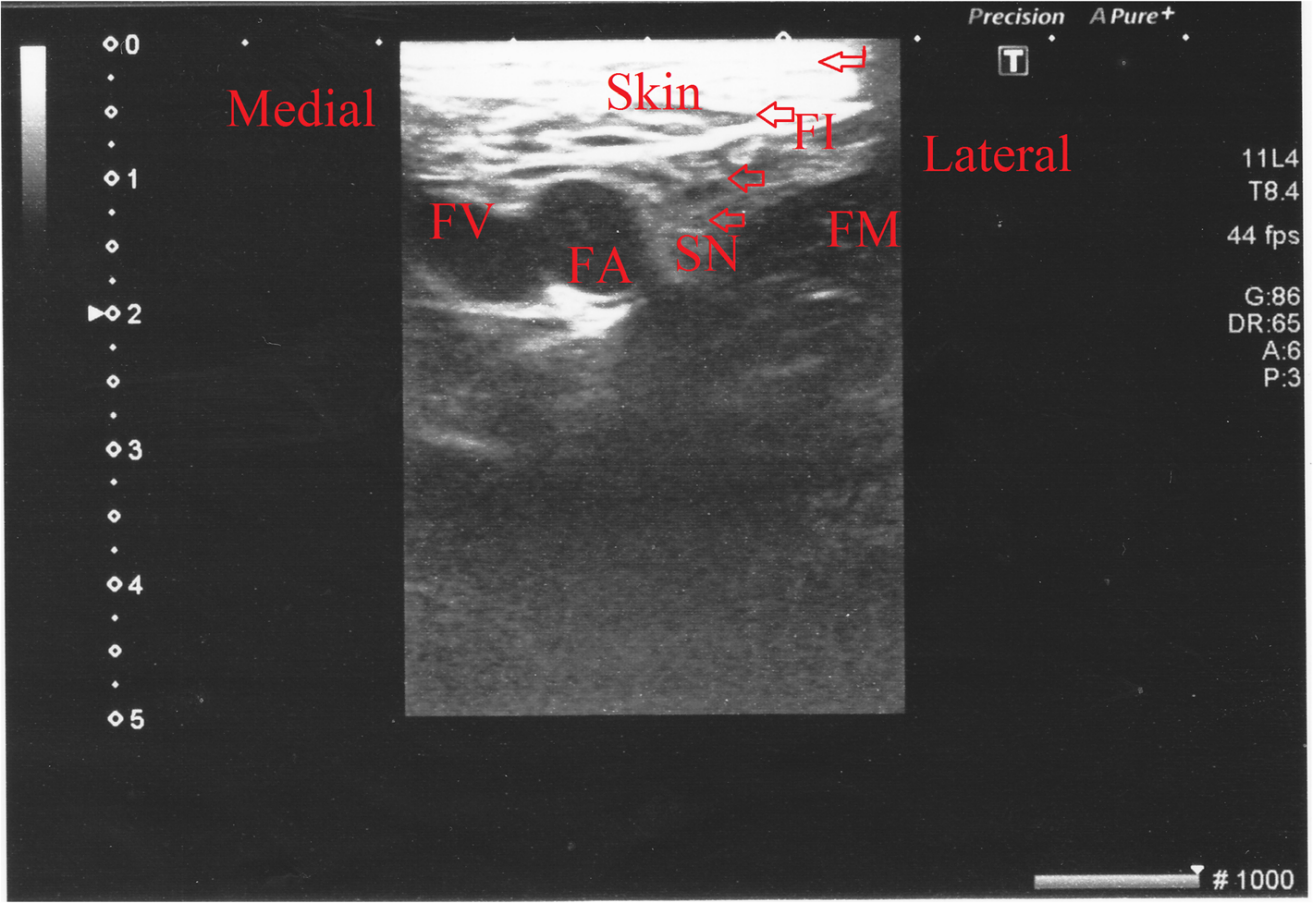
Ultrasound image of the femoral nerve obtained with the anterior approach during the block is shown in the short axis (transverse view). FI: fascia iliaca, IM: liopsoas muscle, LA: local anesthetic, FA: femoral artery, FV: femoral vein, FN: femoral nerve, Arrows: Femoral nerve = needle.
